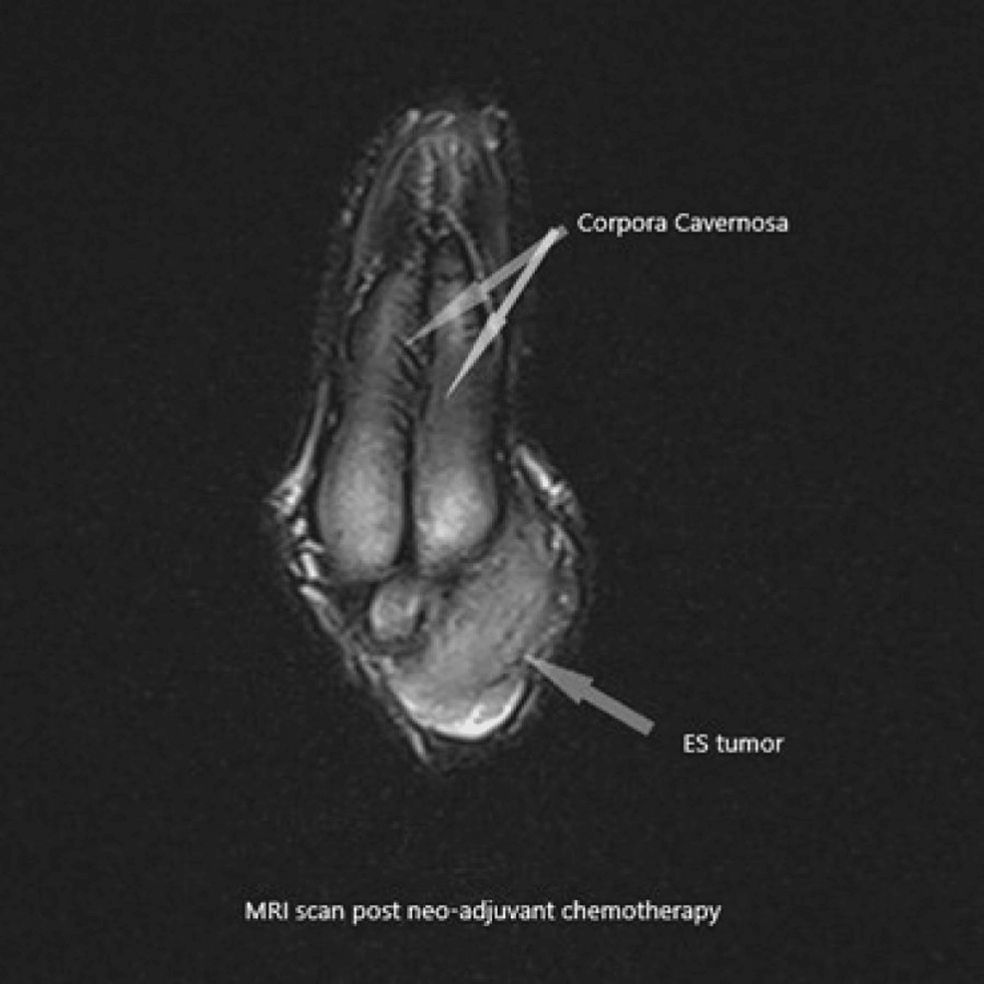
MRI scan post-neo-adjuvant chemotherapy showing interval decrease of the tumor size (coronal plane).
